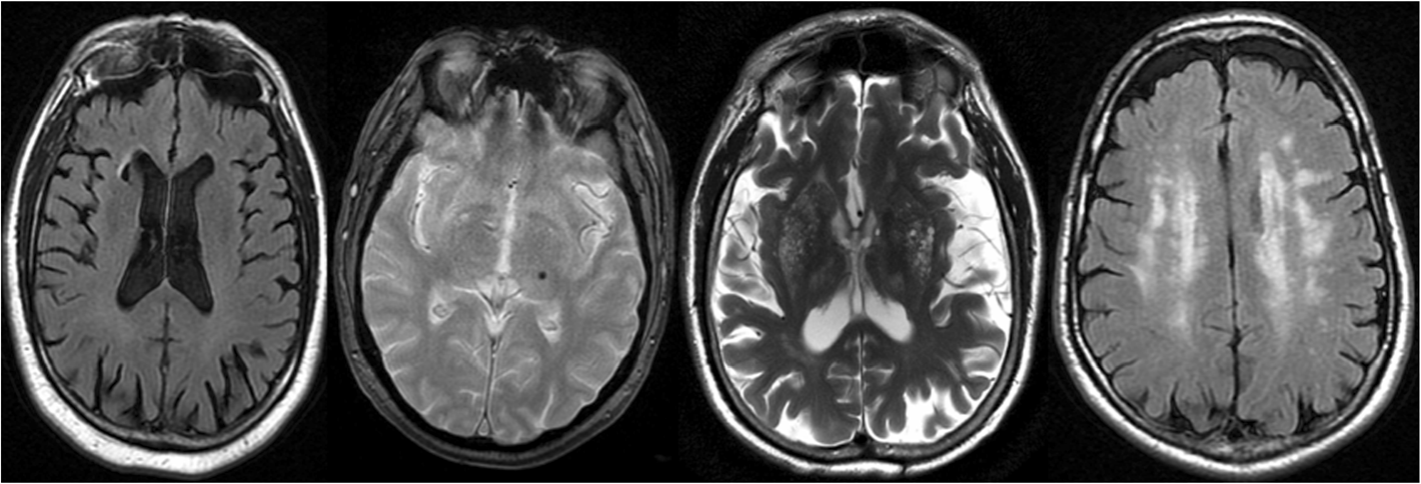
Representative examples of subclinical/silent cerebrovascular lesions. From left to right: Brain infarct affecting caudate nuclei (FLAIR MRI), brain microbleed in left thalamus (GRE MRI), enlarged perivascular spaces involving basal ganglia (T2 MRI) and extensive white matter changes (FLAIR MRI).
